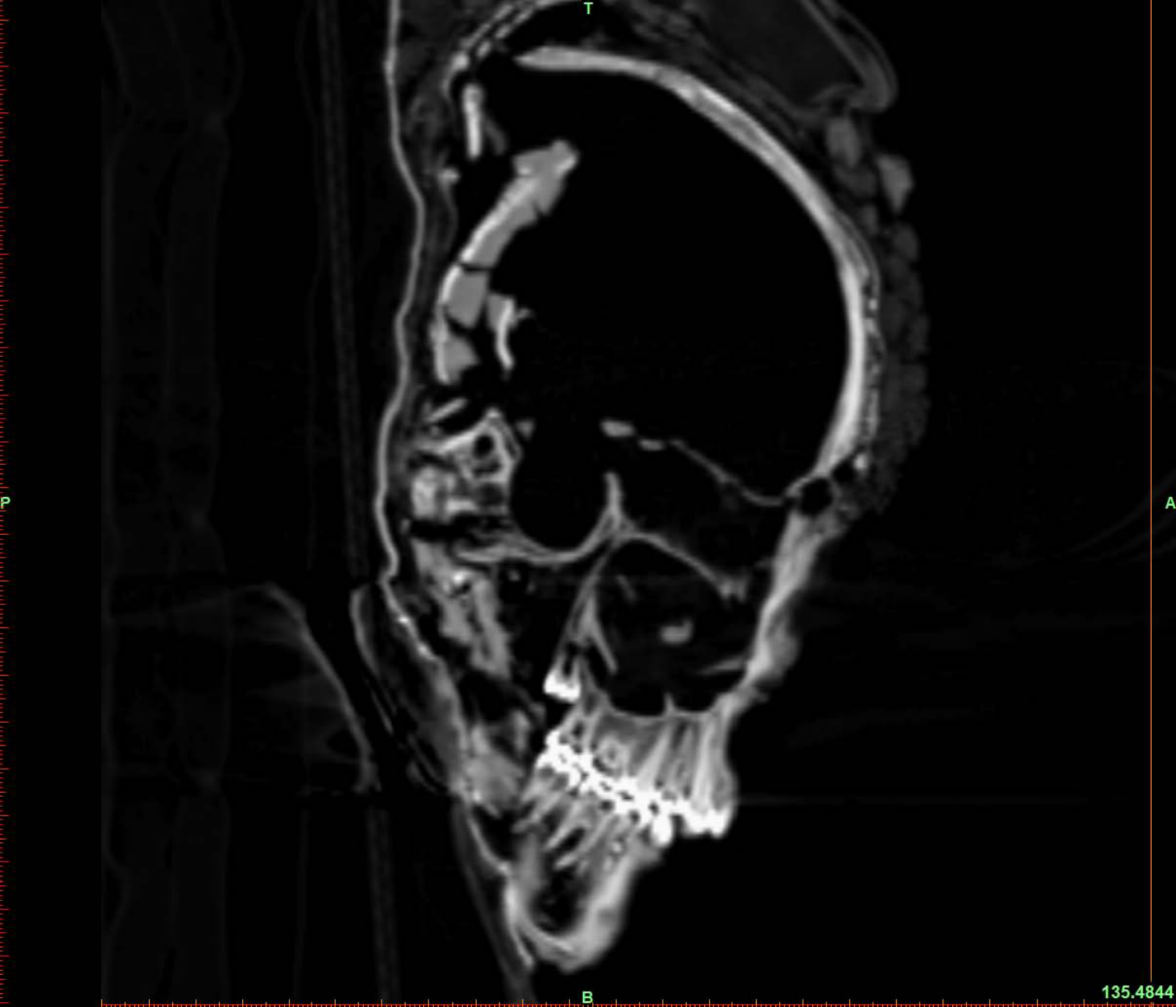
Sagittal CT view of the skull, showing the development state of the teeth.
